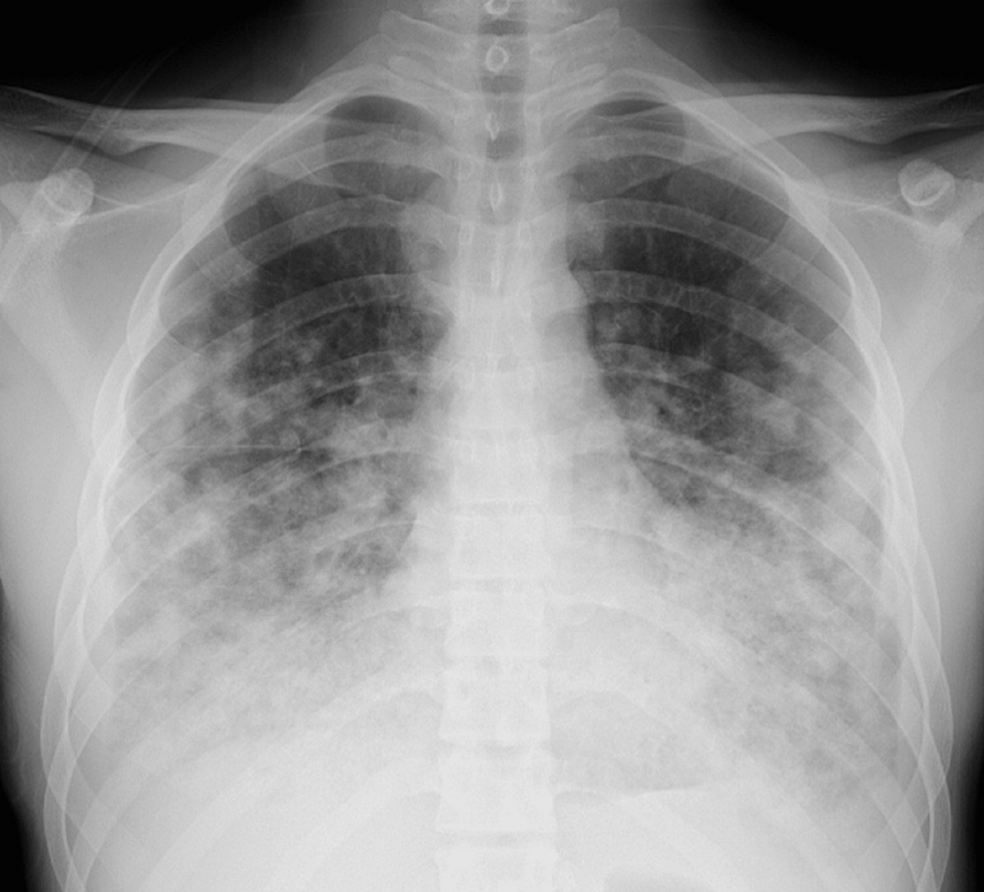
Chest X-ray Chest X-ray on admission showing ground-glass opacities and consolidation in both lower lung fields.
